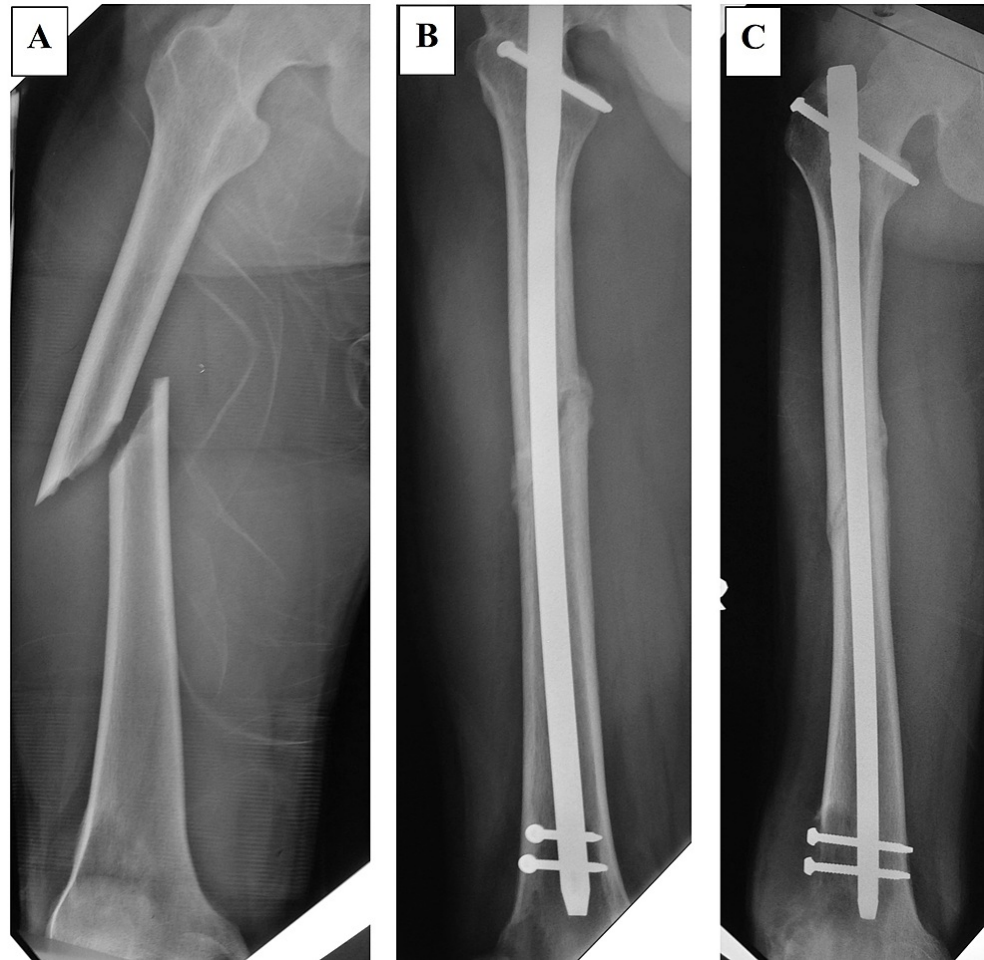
Pre-surgical and three-month post-interlocked nailing plain radiographs The patient sustained a traumatic spiral fracture of the midshaft of the right femur. There was no bony lesion or periosteal reaction seen at the fracture site (A). The fracture had united at three months after surgery with evidence of callus formation (B, C).
